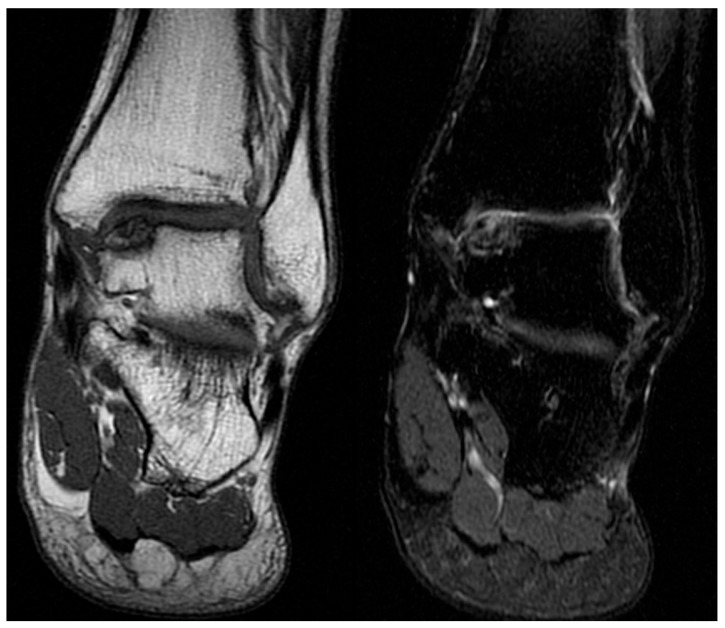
Twelve-year-old male patient presenting with pain and functional limitation in the absence of trauma. MRI reveals osteochondritis dissecans of the medial talar dome.
